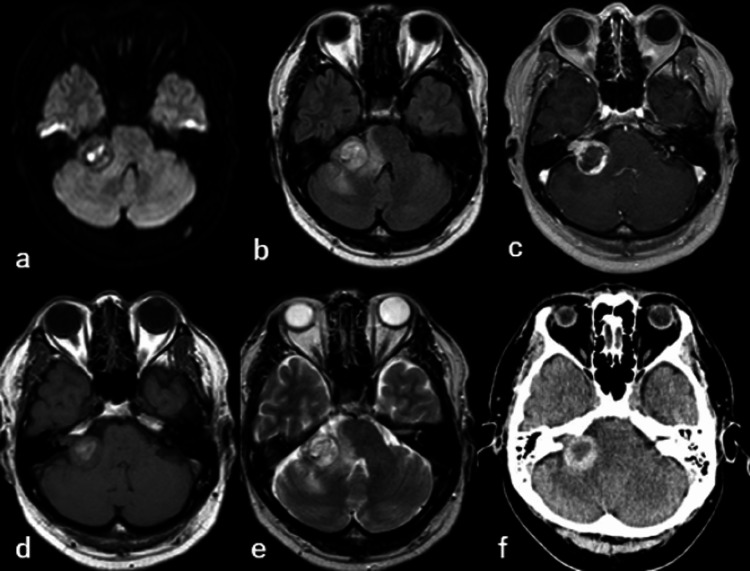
Imaging findings - (a) DWI (b) FLAIR (c) gadolinium-enhanced (d) T1 (e) T2 (f) CT DWI: diffusion-weighted image; FLAIR: fluid-attenuated inversion recovery; CT: computed tomography
